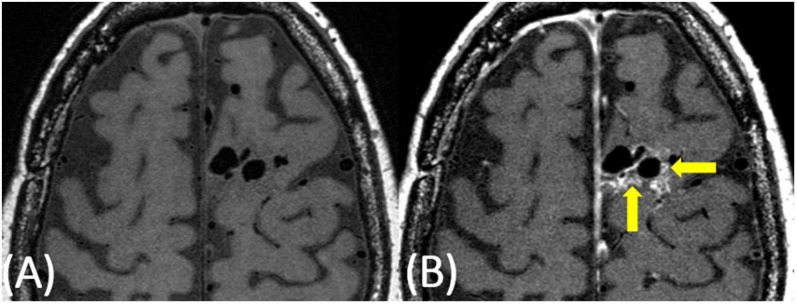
63-year-old female with a predominantly left frontal AVM. On pre-contrast VW-MRI (A), no intrinsic T1 hyperintensity is present. On post-contrast VW-MRI (B), there were multiple areas of hyperintensity at the vessel wall as well as perivascular hyperintensity within the nidus (yellow arrows).
